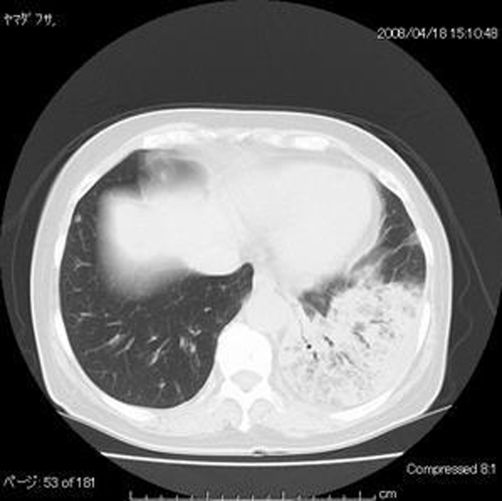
Thin-section CT scan (lung windowing) shows homogenous consolidation with subpleural predominance in the left lower lung.
